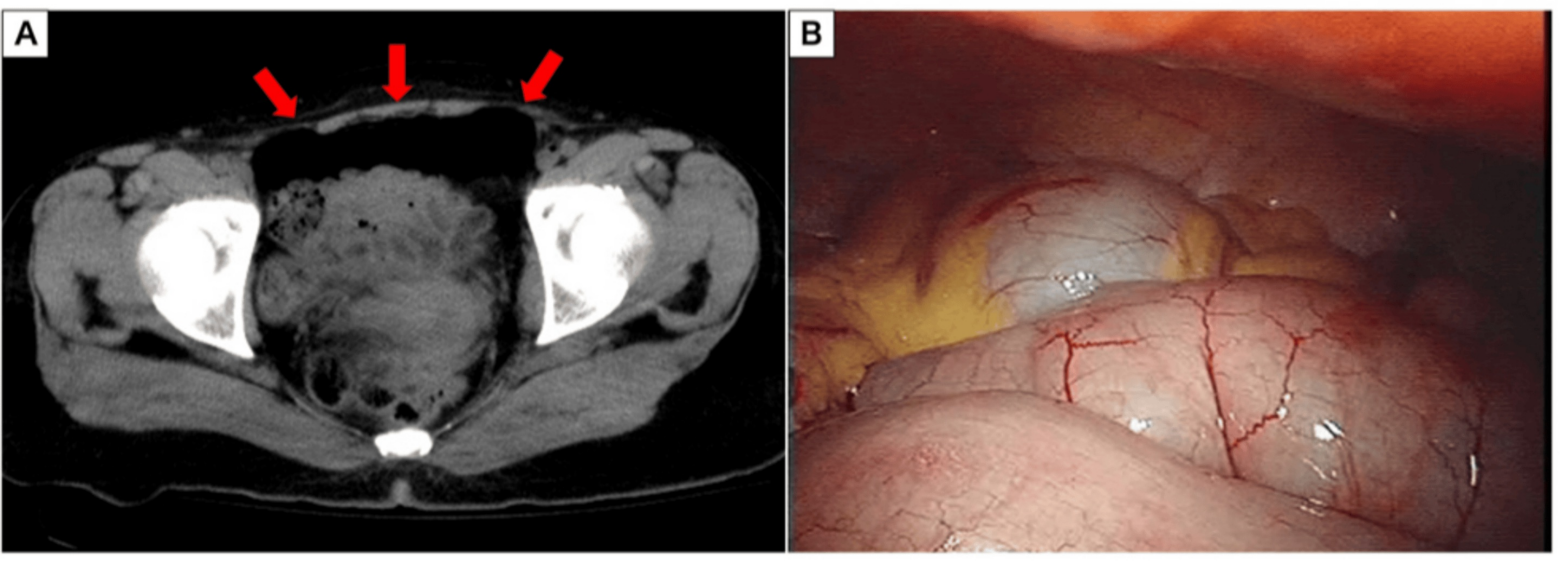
Imaging and intraoperative findings of Case 1. (A) Axial CT image of the lower abdomen showing free intraperitoneal gas (red arrows). (B) Laparoscopic view after a thorough exploration of the peritoneal cavity. No evidence of hollow viscus perforation or contamination was identified.
